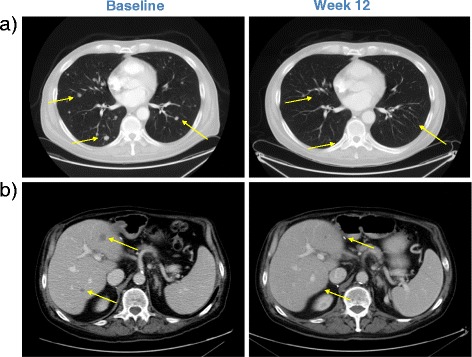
Demonstration of radiographic response to ipilimumab; CT scans performed prior to and 12 weeks after initiation of therapy demonstrate regression of lung and liver metastases (arrows).
